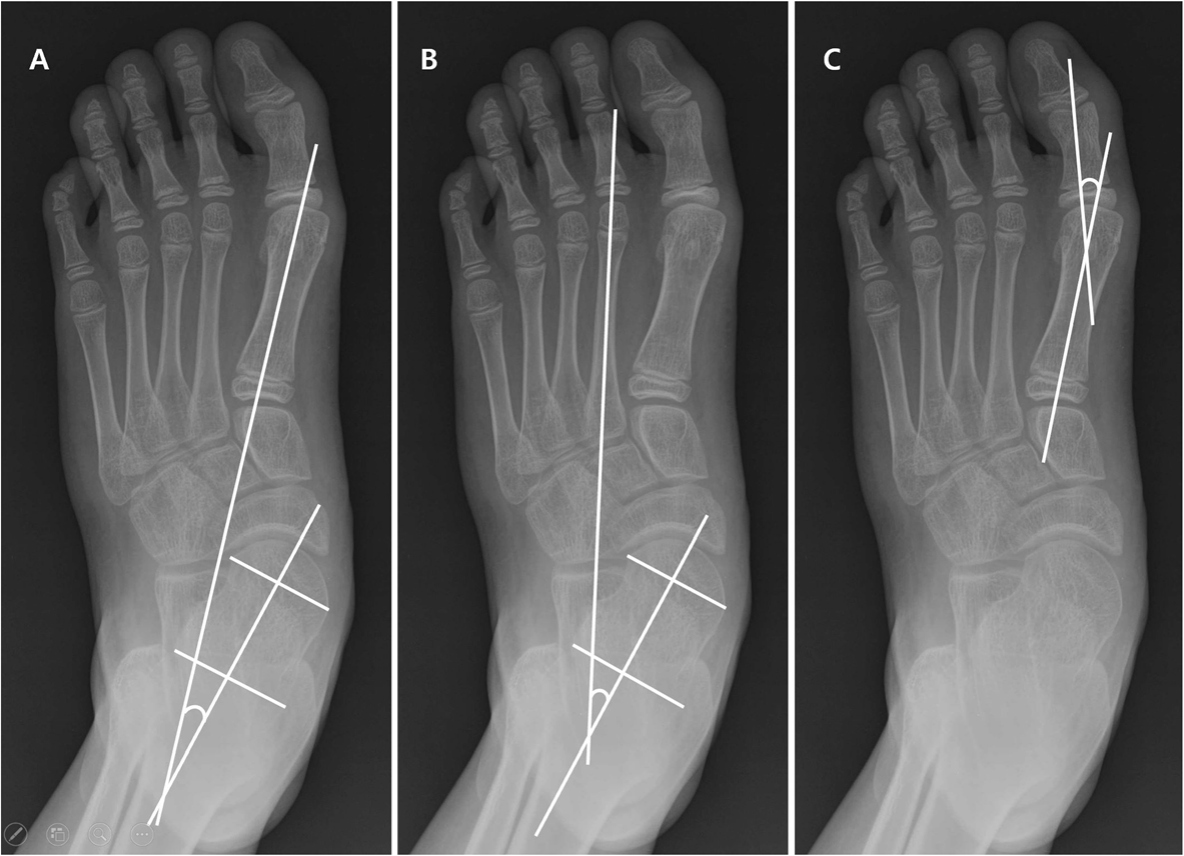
a The anteroposterior talo-first metatarsal angle is the angle between the line bisecting the longitudinal axis of the first metatarsal bone and the line bisecting the longitudinal axis of the talus on standing anteroposterior plain foot radiograph. b The anteroposterior talo-second metatarsal angle is the angle between the line bisecting the longitudinal axis of the second metatarsal bone and the line bisecting the longitudinal axis of the talus on standing anteroposterior plain foot radiograph. c The hallux valgus angle is the angle between the longitudinal axis of the first proximal phalanx and the longitudinal axis of the first metatarsus
